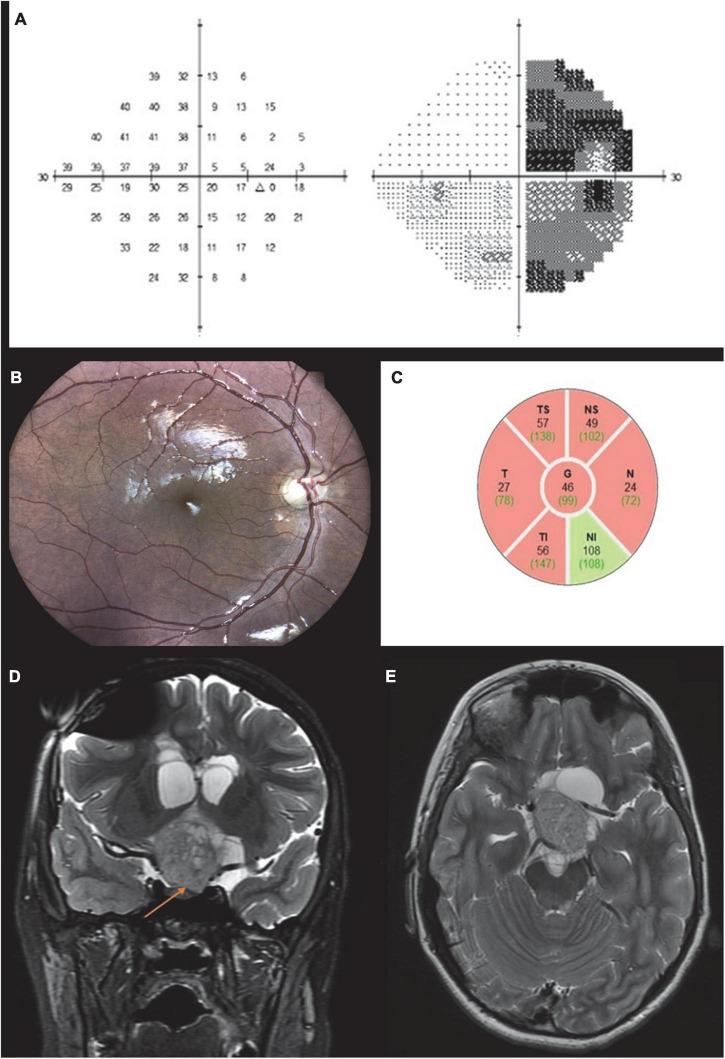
Case 4. Automated perimetry demonstrating temporal hemianopia in the right eye (A). Brain MRI T2 coronal (B) and axial images (C) showing a large chiasmatic lesion extending to the left optic tract and left pre chiasmatic optic nerve causing compression of the third ventricle. Fundus color photograph shows bow tie atrophy of the right optic disc (D). OCT scan of the right eye showing diffuse RNFL thinning (E).
